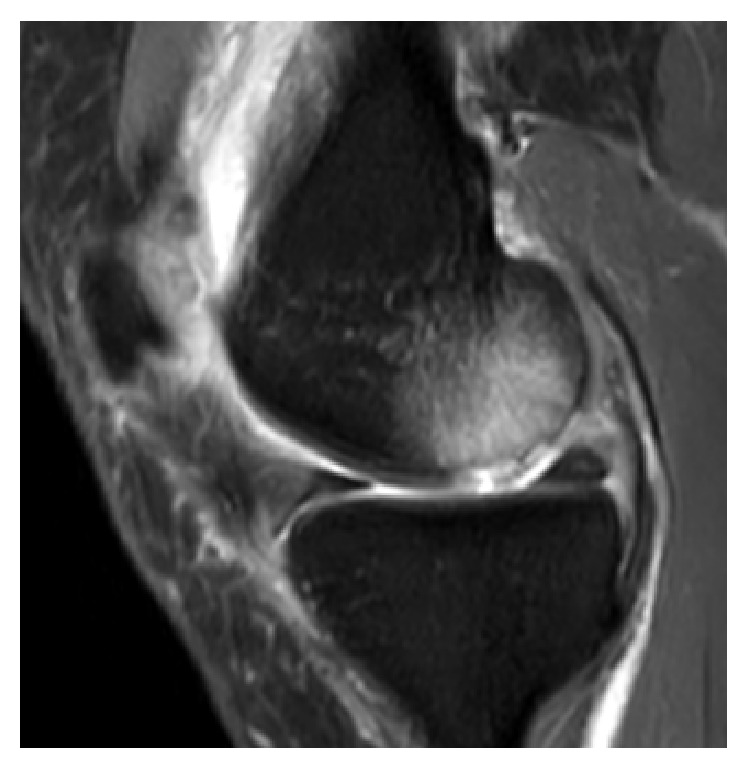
Sagittal T2-weighted MRI of left knee joint depicting cartilage lesion and large underlying BME at dorsomedial femoral condyle.
